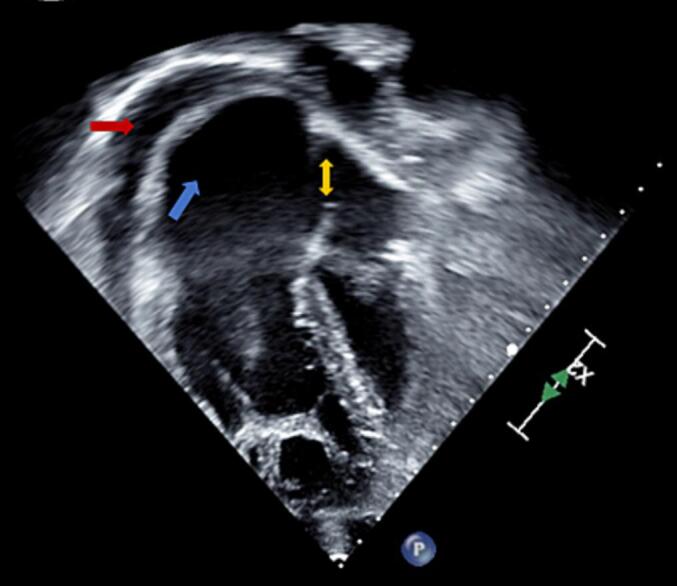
Congenital transthoracic echocardiogram (TTE) obtained after repeat pericardiocentesis. This figure shows congenital TTE obtained after repeat pericardiocentesis, revealing a moderate pericardial effusion (red arrow), severely enlarged right atrium (blue arrow) and atrial septal defect (ASD) (yellow arrow).
